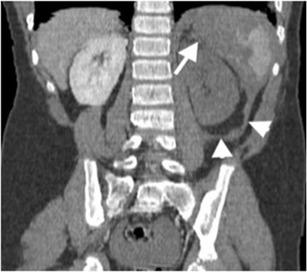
Splenic subcapsular hematoma. Coronal contrast-enhanced CT image of a 18-year-old man demonstrates parenchymal, subcapsular splenic hematoma (*arrow*) and accompanying retroperitoneal hematoma (*arrowheads*). Devascularised left kidney is also noted
